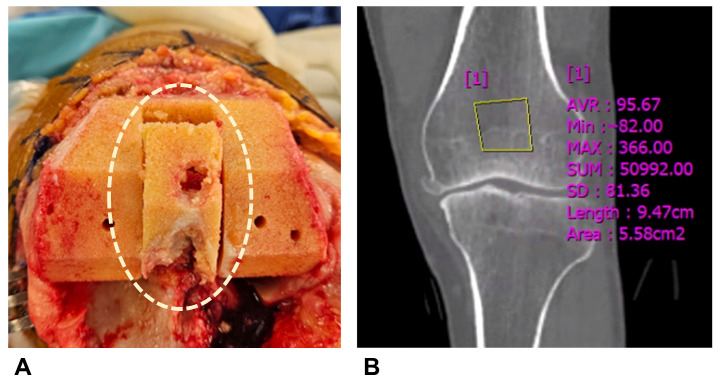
Hounsfield unit measurement. (A) Box bone area where Hounsfield unit was measured. (B) Hounsfield units were measured by setting rectangular regions of interest in the box bone area on CT coronal images.
